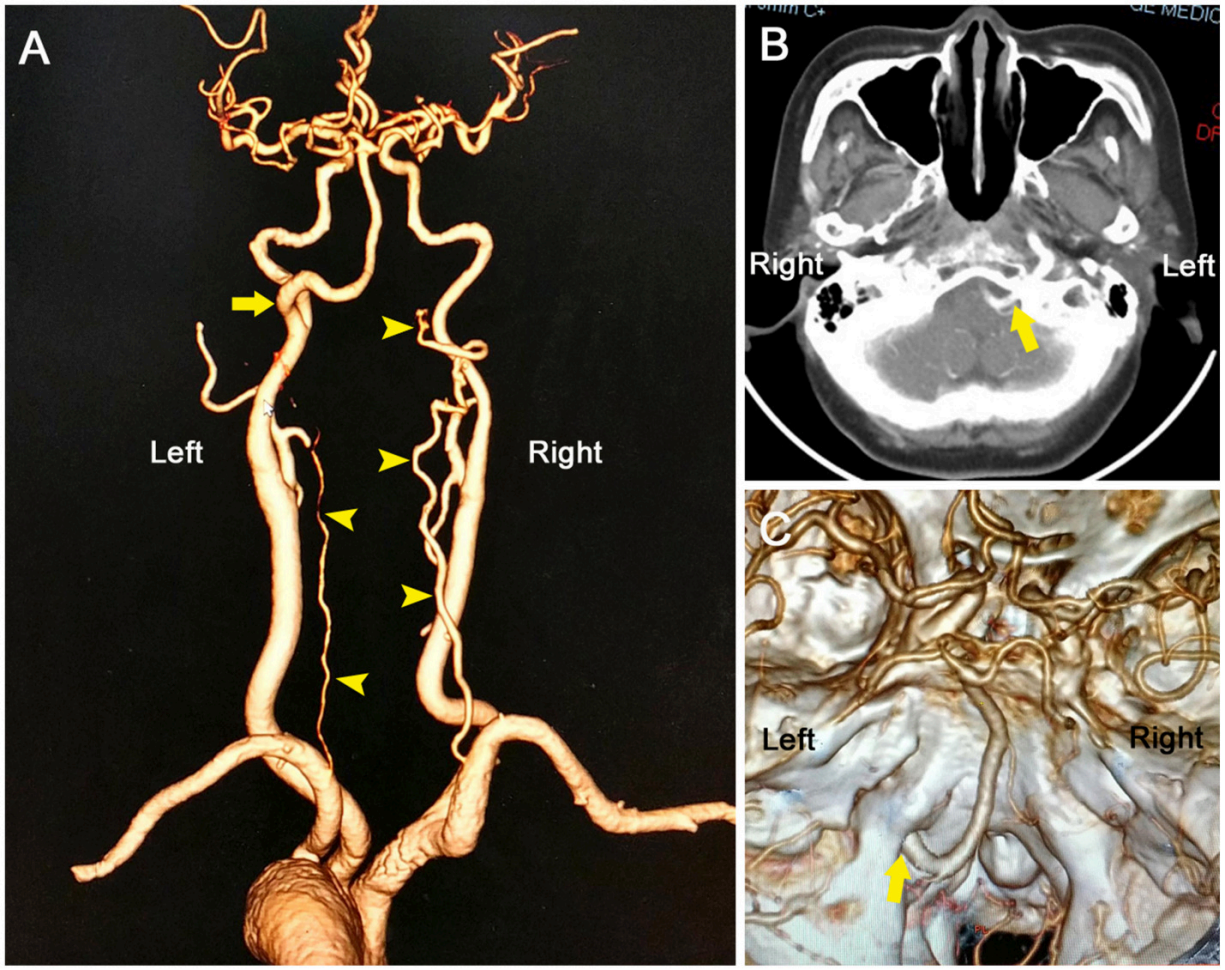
Computed tomography angiography showing an anomalous vessel (A, arrow) arising from the left internal carotid artery and then traveling through the left hypoglossal canal (B,C), consistent with the diagnosis of persistent hypoglossal artery. Note that bilateral vertebral arteries were hypoplastic (arrowheads), and no posterior communicating arteries were found; therefore, the PHA primarily fed the posterior circulation.
